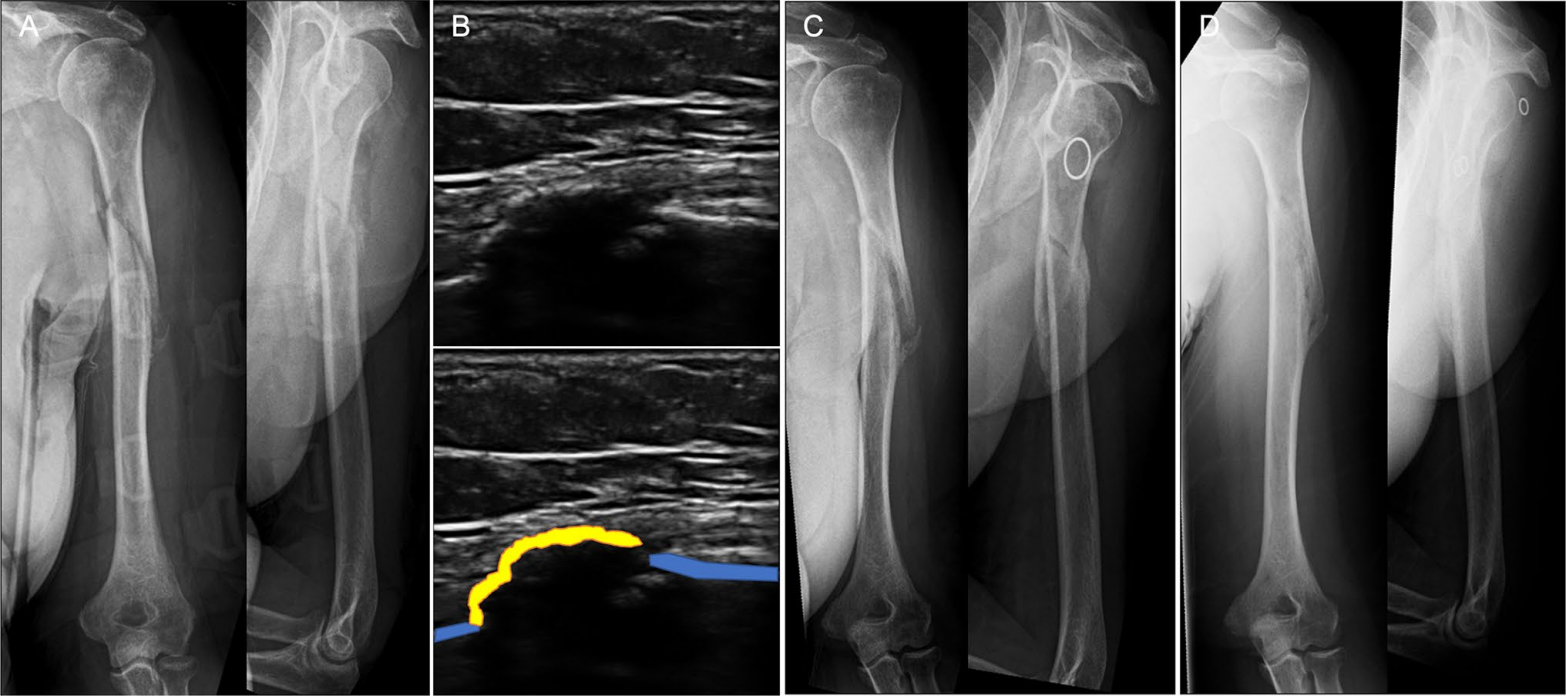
Sequence of images for a 59 year-old female patient with a left humeral shaft fracture: A Six-week anteroposterior (AP) and lateral radiographs (Radiographic Union Score for HUmeral fractures = 7); B Six-week ultrasound scan image (blue = bone cortex, yellow = sonographic callus); C 12-week AP and lateral radiographs (showing union); D Eight-month AP and lateral radiographs (showing fracture consolidation)
